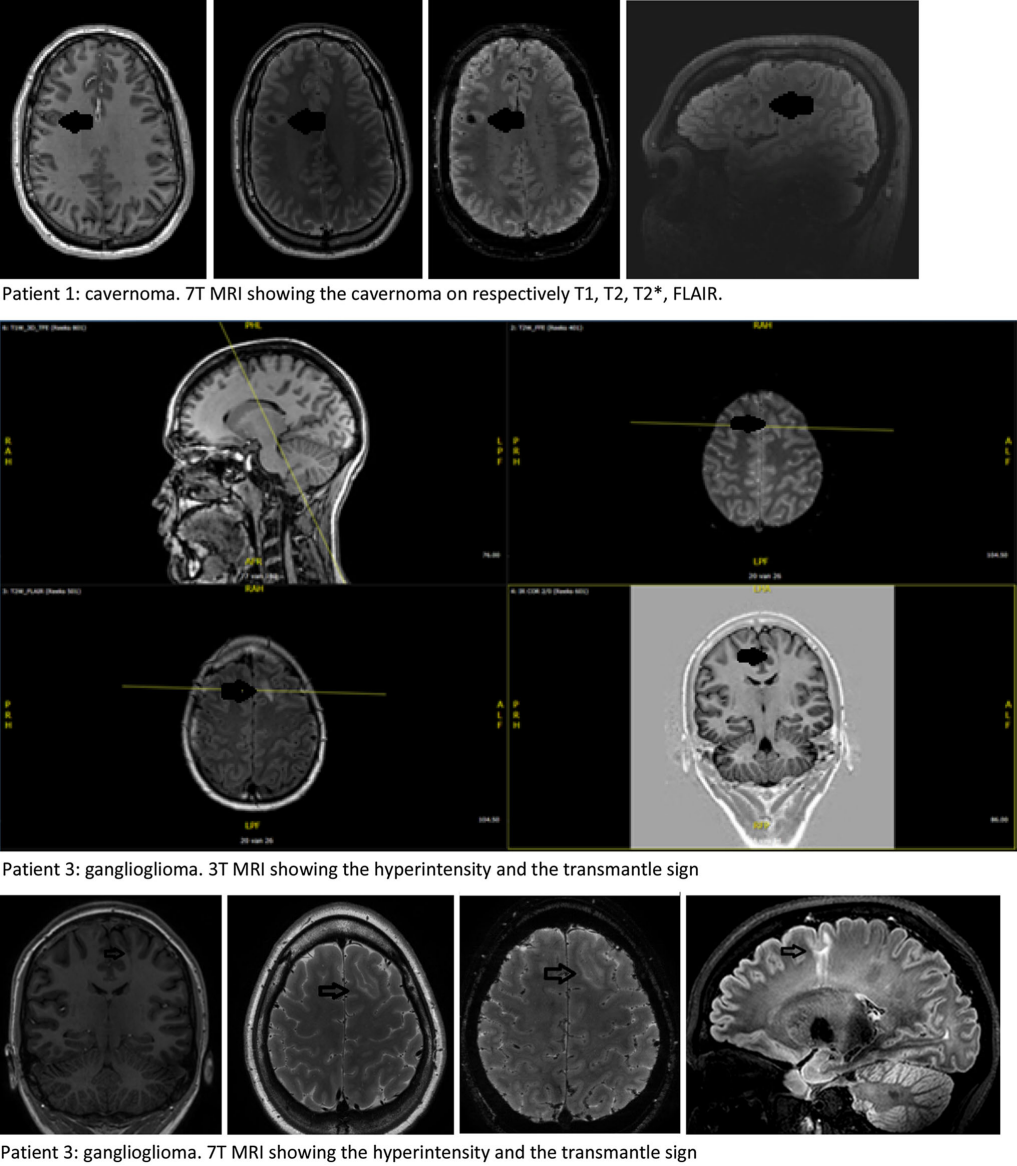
3 and 7 T MRI images of the two patients with changed diagnosis. Each sequence contrasted and angulated individually
